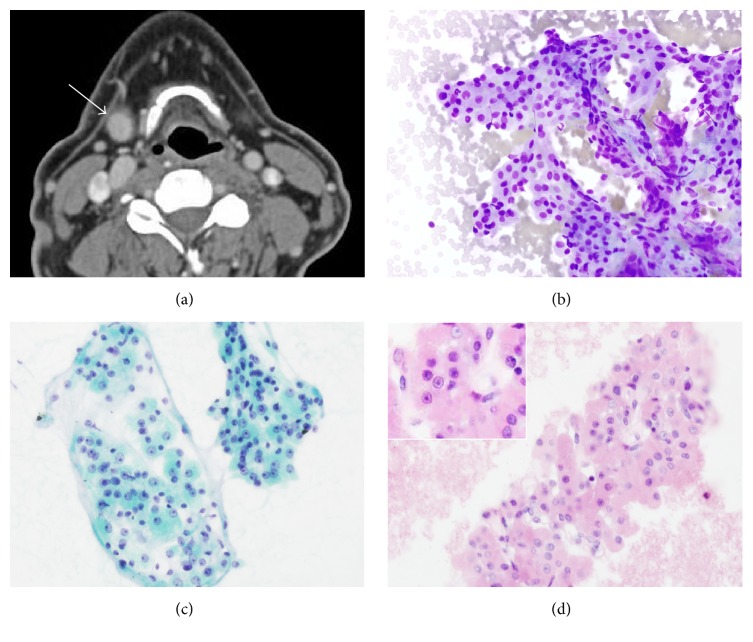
Imaging and cytopathology of the submandibular oncocytoma. (a) CT scan showing a well-circumscribed mass (1.6 × 1.3 cm) at the right submandibular space; (b)–(d) FNA of the mass showing clusters of polygonal eosinophilic epithelial cells with low N/C ratio, round nuclei, and prominent nucleoli ((b) Diff-Quik, ×400; (c) pap smear, ×400; and (d) cell block, ×400 (inset, ×600)).
